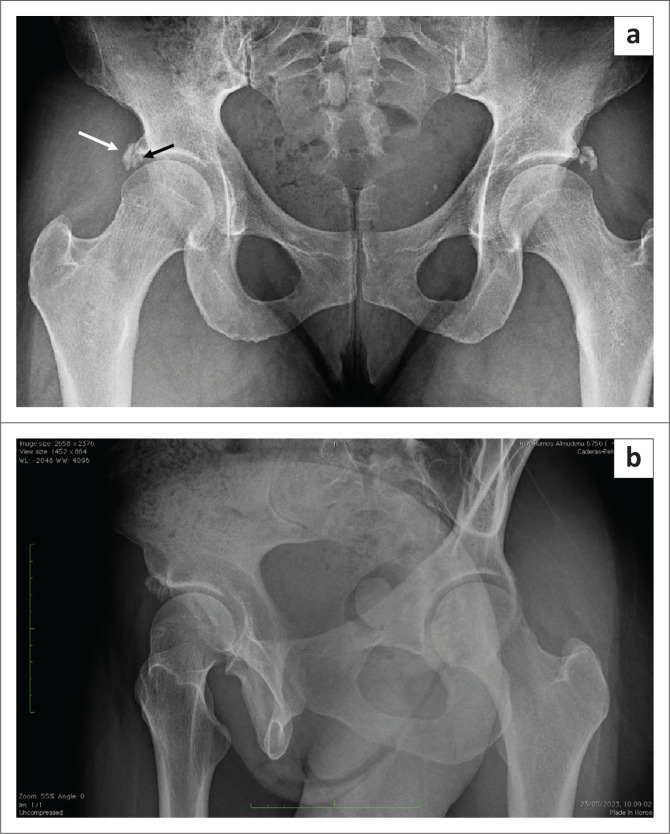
(a) Anteroposterior pelvis radiograph. Double calcifications are seen pre-operatively at the superolateral edge of both acetabula, separated by a subtle radiolucent line and a small subarticular cyst. The calcification of the rectus femoris originating from the anterior inferior iliac spine is denoted on the right hip by the solid white arrow, while the punctate calcifications of the acetabular labrum is denoted by the solid black arrow. (b) C Profile view revealing the labral and the rectus calcifications, a synovial herniation pit and the cam morphology of the femoral head.
